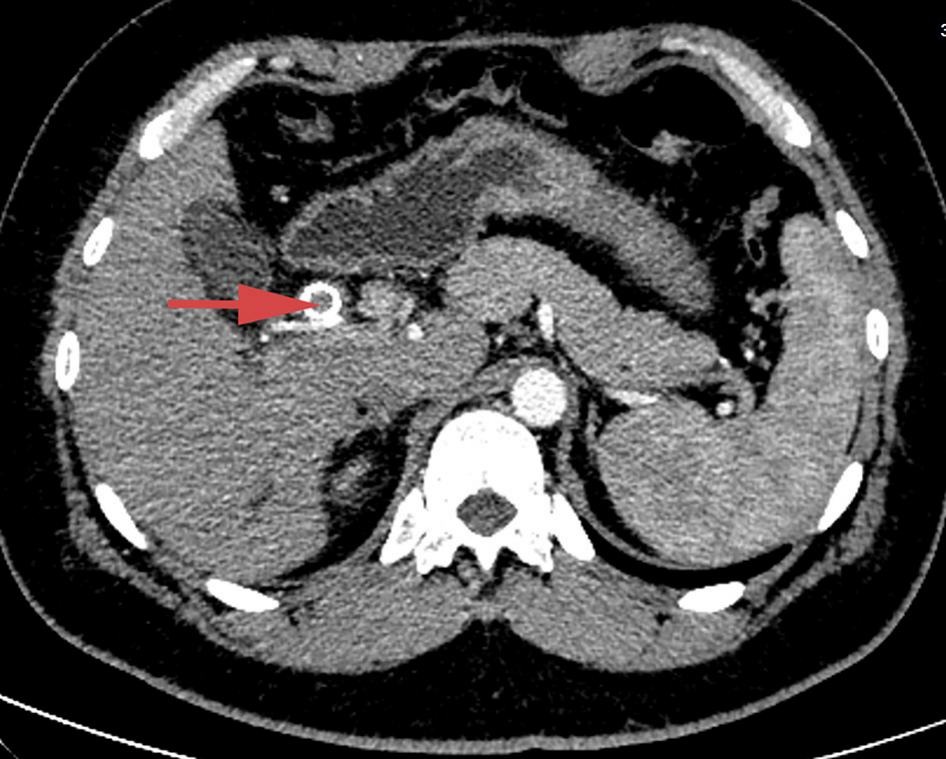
Abdominal contrast-enhanced CT scan, showing high-density shadow in the initially placed biliary metal stent and lumen obstruction.
